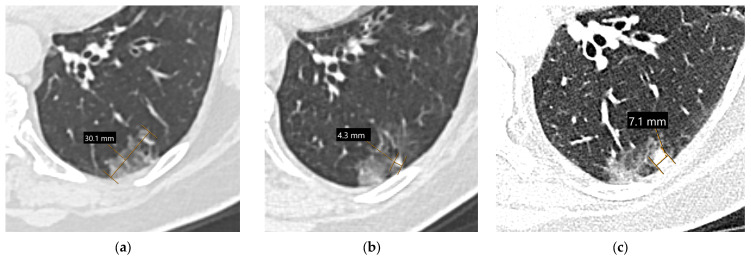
Measurement of subsolid nodule diameter in the lung window setting (width 1500, level −600; the same patient as in Figure 1c): The recommended diameter measurement of the pure ground-glass nodule on chest CT requires measurement of its longest diameter and is classified as Tis if the diameter is between 0.5 cm and 3 cm (a). Two years later, the same lesion is partially solid, with the largest dimension of the solid component being 4 mm; therefore the lesion should be categorized as a minimally invasive adenocarcinoma (T1mi, (b)). Three years later from the basal scan, the solid component has a diameter of 7 mm (c); however, due to visceral pleural invasion, it should be classified as T2a (adenocarcinoma with visceral pleural invasion was confirmed pathologically after lower-left lobectomy).
